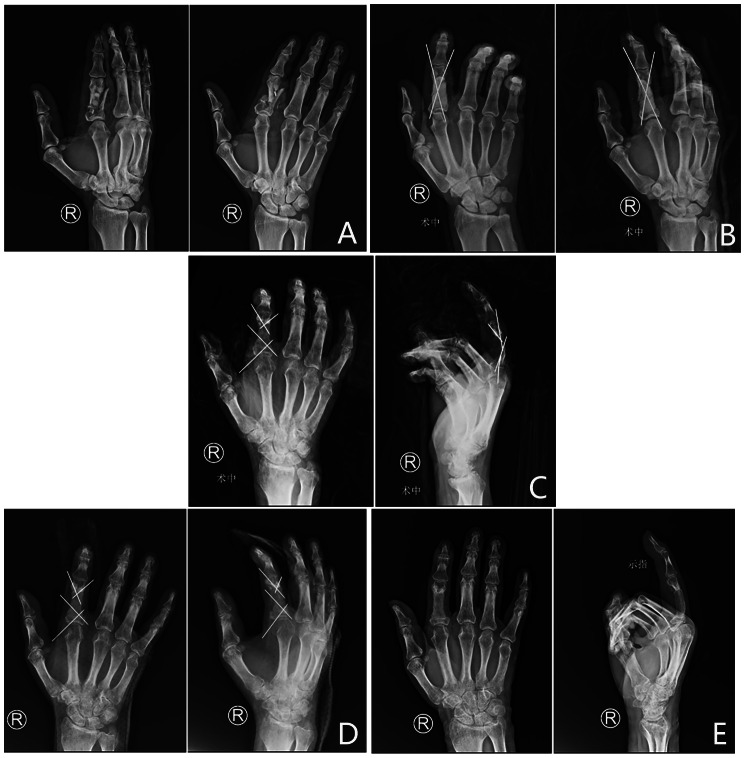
The right index finger of a 56-year male patient was impaired by a roller machine, resulting in open fracture of the proximal phalanx with tendon, nerve, vessel, and soft tissue defects. A, Preoperative X-ray. B, After debridement, the proximal phalanx presented a segment defect, and cement shaped like the phalanx was used to fill the space. C, At 8 weeks postoperatively, the cement spacer was removed, and the bone graft was carved into a 2.0 cm ⋅ 1.2 cm ⋅ 1.0 cm cylindrical shape similar to the shape of the metacarpal. Two crossed Kirschner wires were used to fix the fracture. D, At eight weeks postoperatively, callus formation was observed. E, At 8 weeks postoperatively, bone graft union was observed without additional infection
